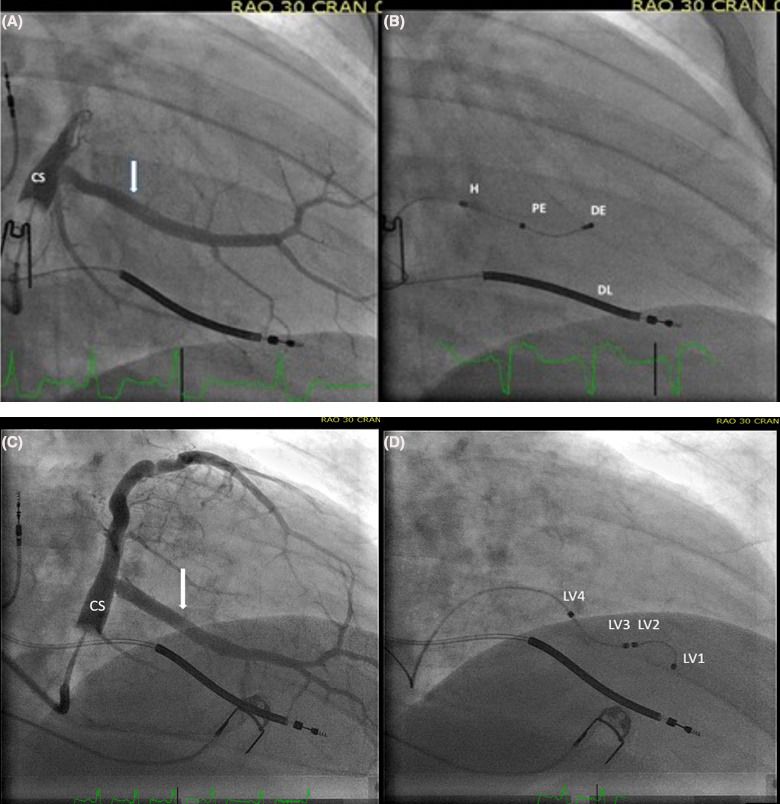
Right anterior oblique fluoroscopic views of two patients with an active fixation bipolar lead (A + B) and passive fixation quadripolar lead (C + D). On the coronary sinus (CS) venograms (A + C) the arrows indicate the target veins in lateral side branches from CS. The target vein is located in the target left ventricular segment determined from speckle tracking echocardiography. B: The final lead placement of an active fixation bipolar lead. The helix (H) is fixated proximal in the vein. The proximal electrode (PE) is located in a basal third left ventricular long‐axis position, and is used as the stimulating cathodal electrode. The distal electrode (DE) is in the mid third left ventricular long‐axis segment. The high voltage right ventricular defibrillator lead (DL) is located close to the apex of the right ventricle. D: The final lead placement of a quadripolar lead. The distal end (LV1) is wedged into a small side branch. The proximal electrode (LV4) is used as the stimulating cathodal electrode
